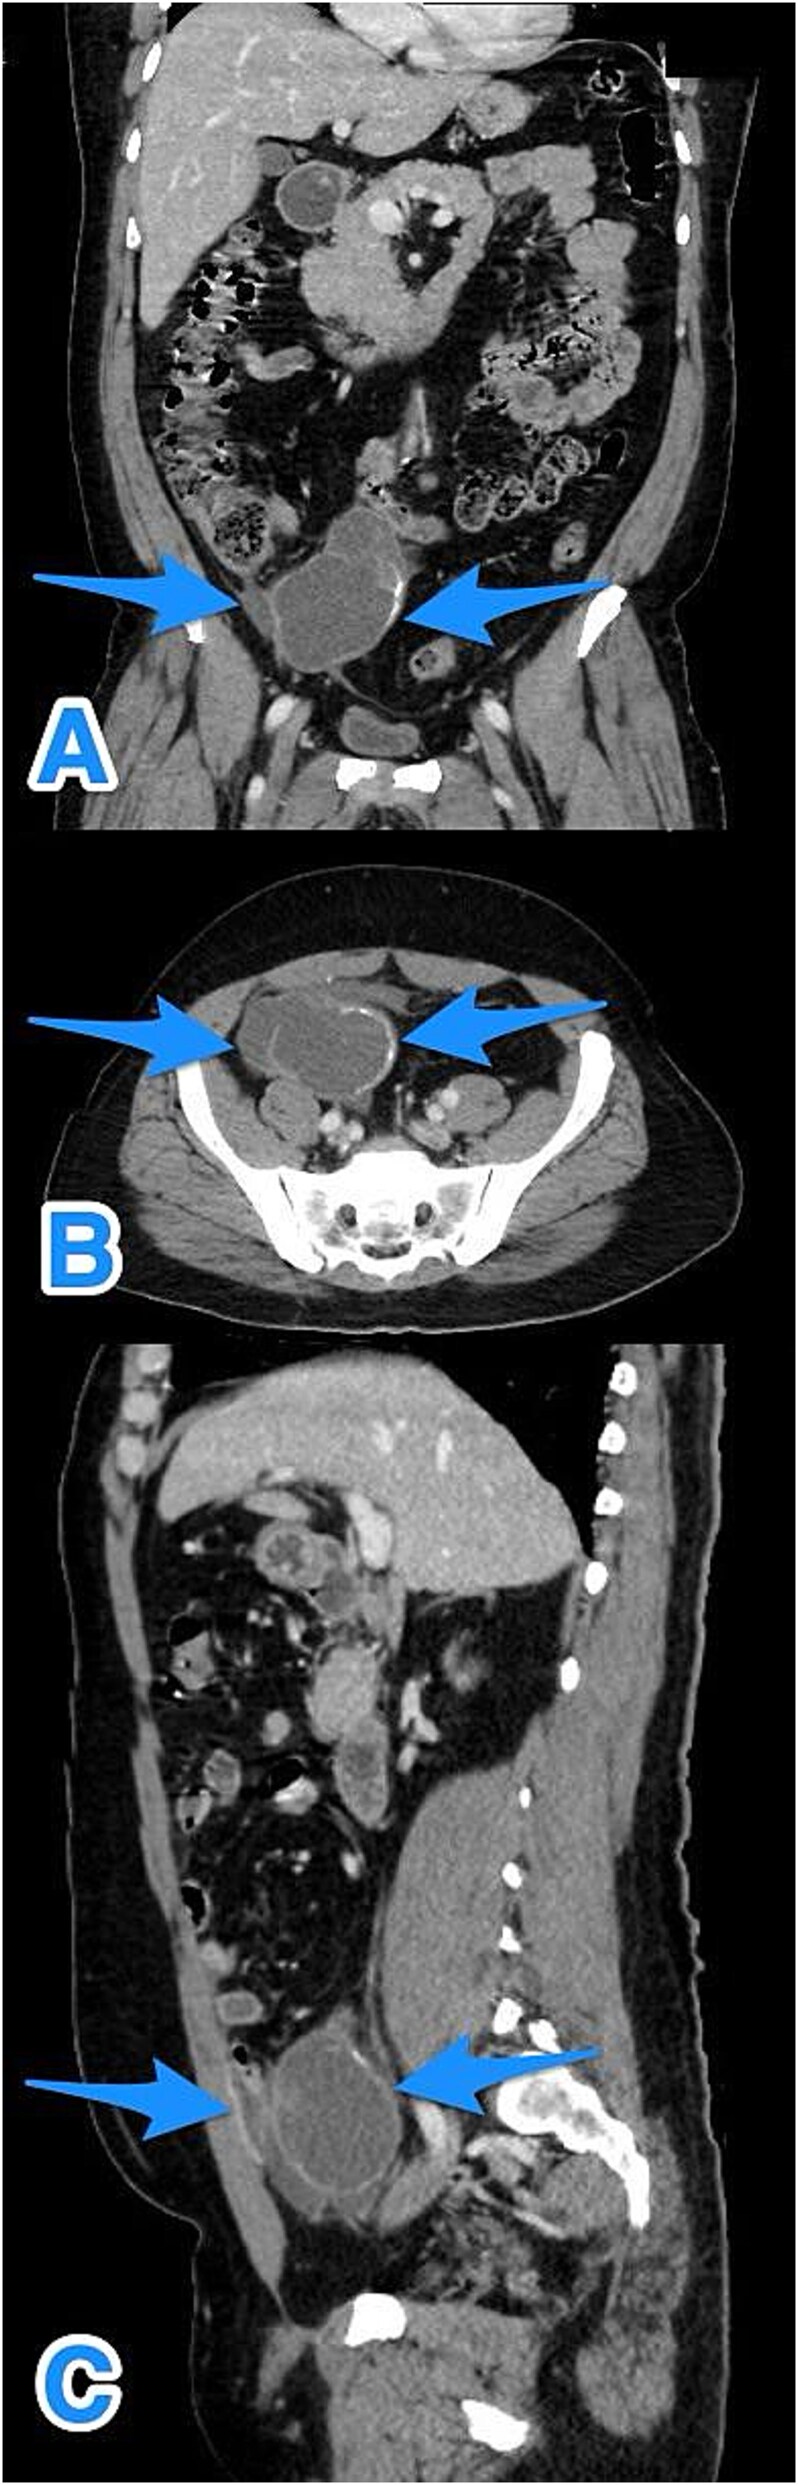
Multiview contrast-enhanced CT images of the perforated appendix with associated free fluid: (A) coronal view, (B) axial view, and (C) sagittal view (arrows).
